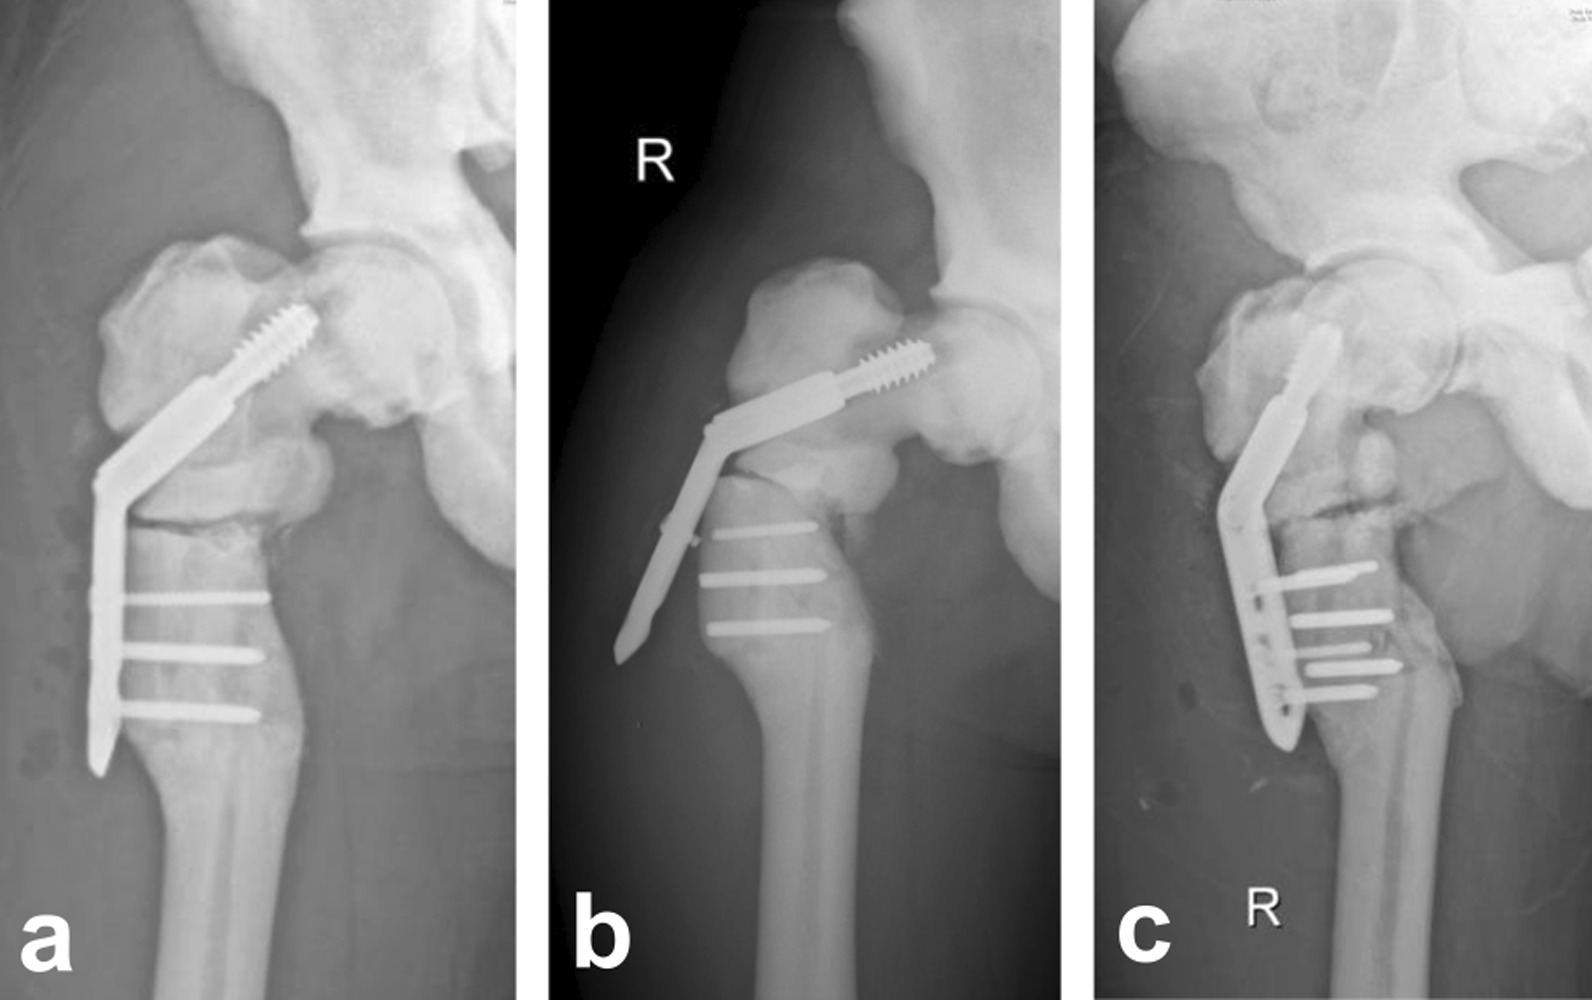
X-rays. a 1 week after the first operation. b Screw fracture 3 months after the first operation. c 2 days after the repeated DHS fixation
